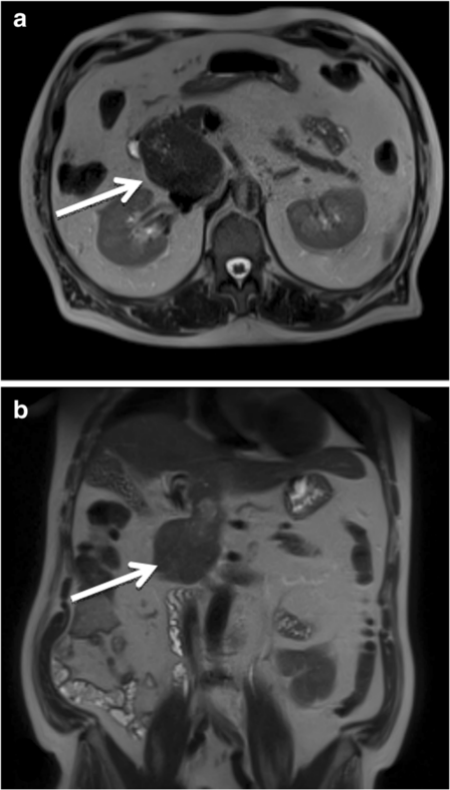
MRI scan of the discovered mass which compresses the large intraabdominal vessels (arrow)1a transverse section of the tumor 1b frontal section
